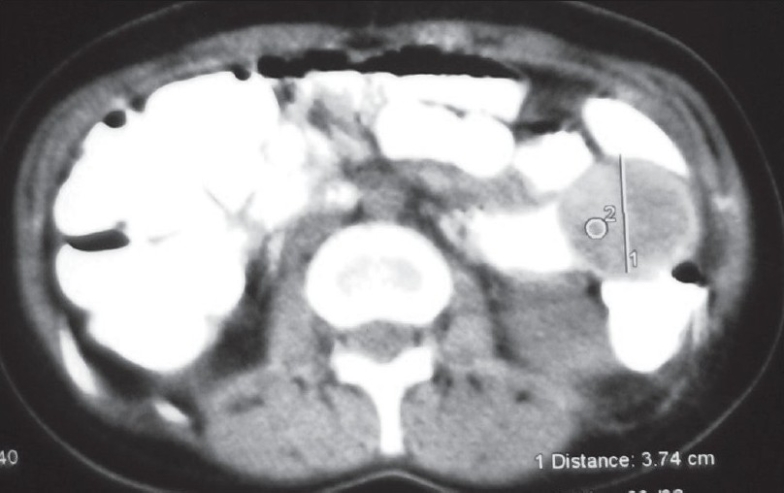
Another section of the contrast-enhanced abdominal CT scan with oral contrast administration revealed an ovoid and well-defined, low-density tumor in the intussusception
